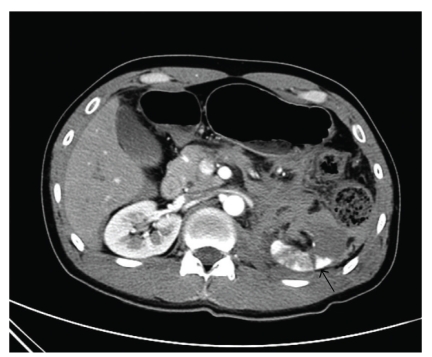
Computed tomography after abdominal trauma reveals left renal injury (grade IV, black arrow) and perirenal hematoma (white arrow).
